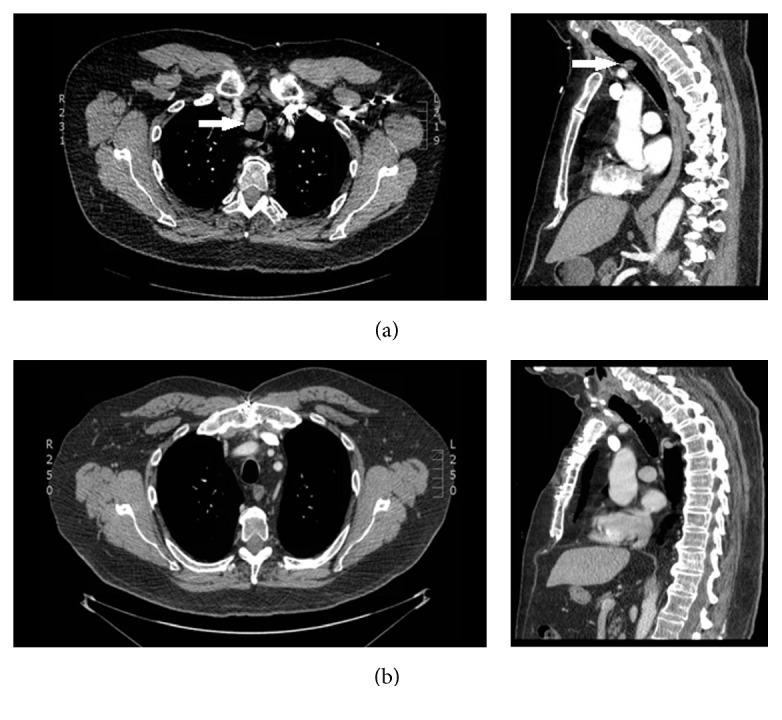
CT scan preoperative axial and sagittal views of the tumor (white arrows, (a)); postoperative views of resected trachea (b).
